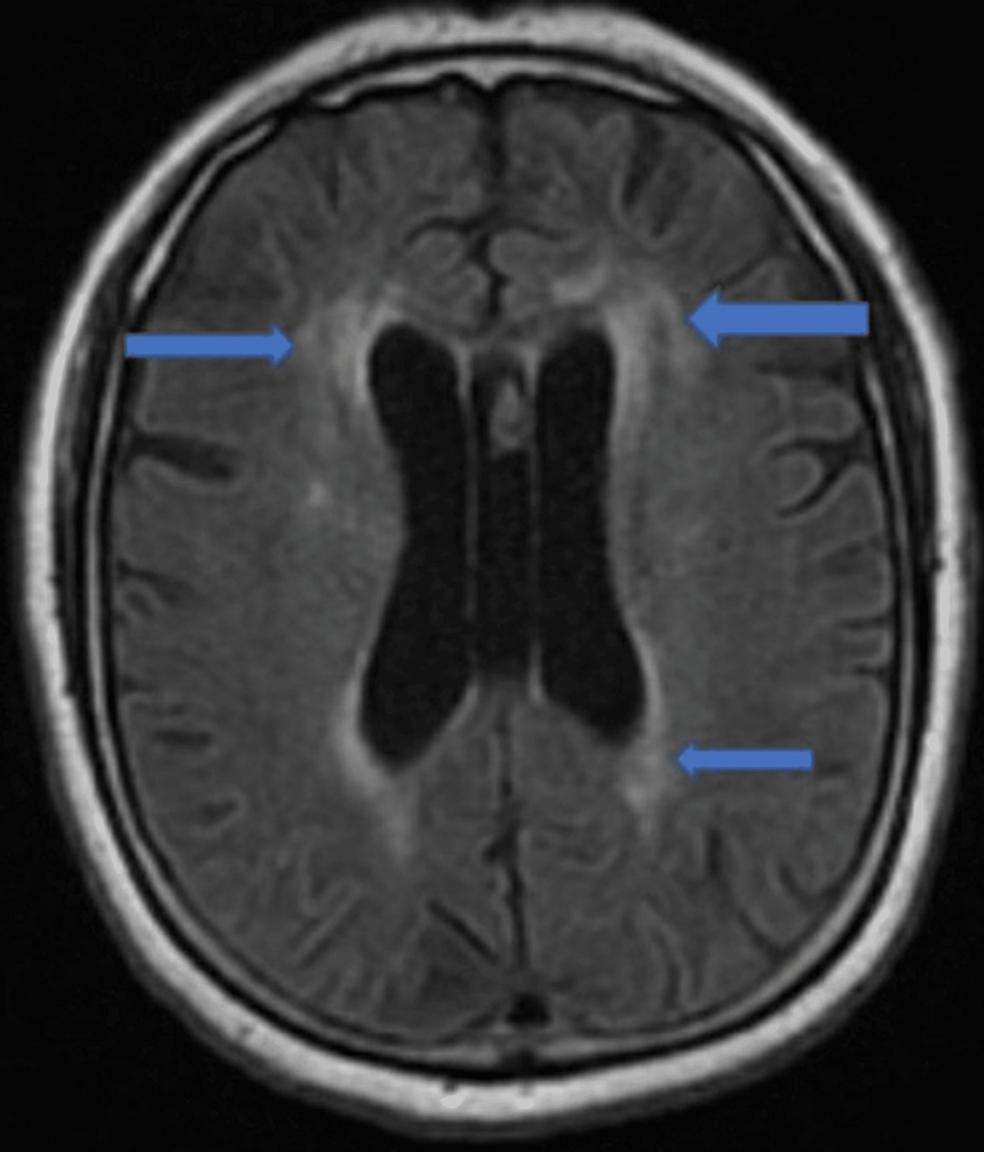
MRI brain showing bilaterally increased signal intensity (blue arrow)
